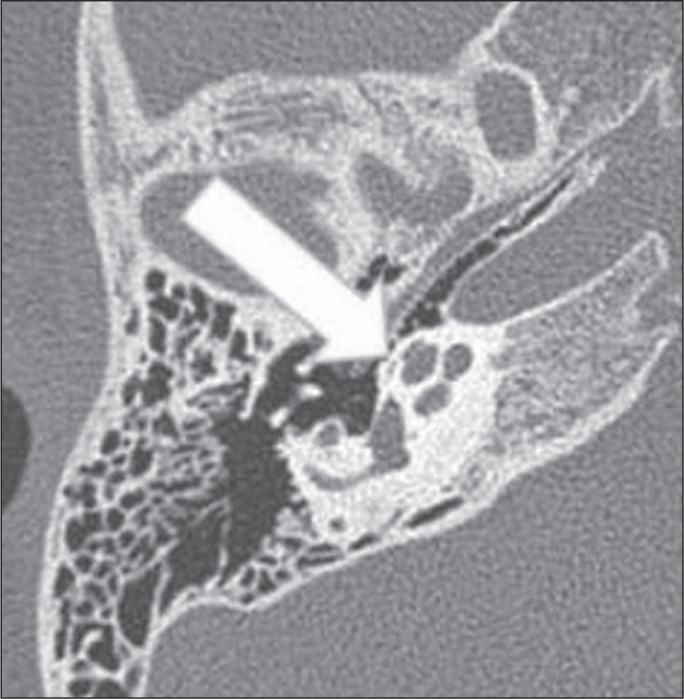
Cochlear cleft in a 15-year-old adolescent male. Unenhanced axial CT
